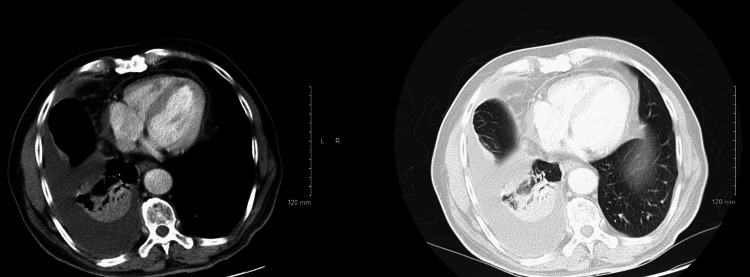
CT chest with contrast evident for right pleural effusion and basilar atelectasis and/or consolidation.
